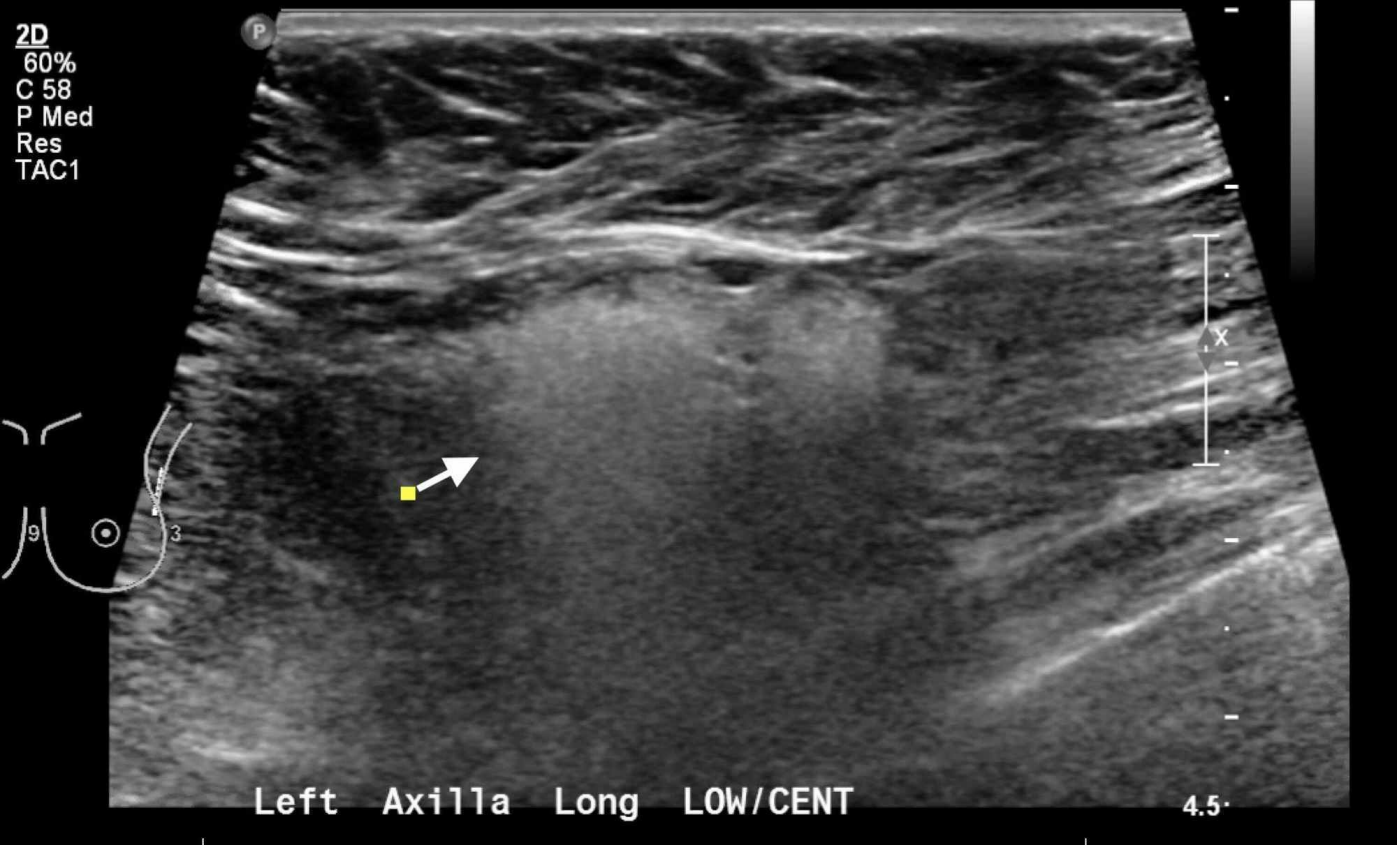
Preoperative ultrasound 2D ultrasound showing two left axillary lymph nodes with "sandstorm" appearance (white arrow) consistent with silicone lymphadenopathy
